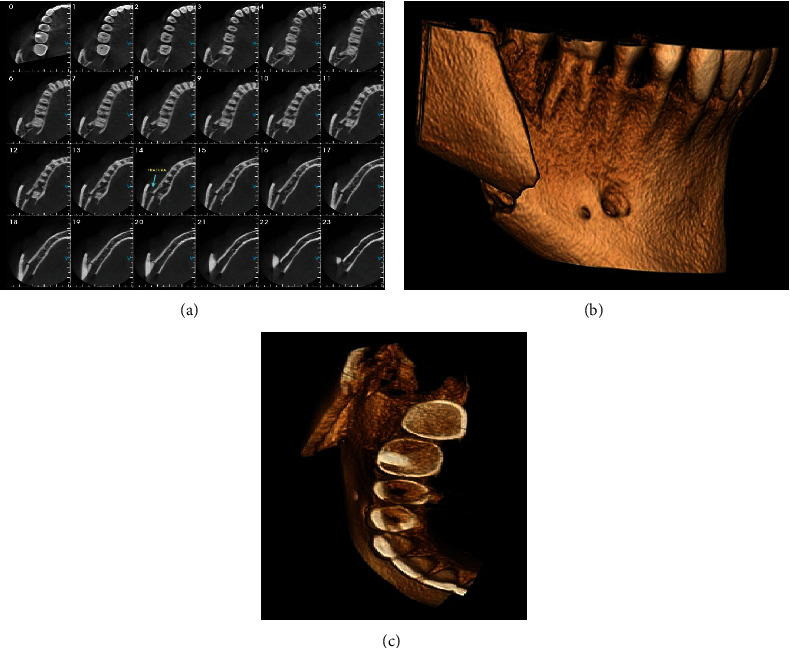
(a) Axial cuts showing right mandibular body fracture. Three-dimensional reconstruction of CT scan showing fracture of the right mandible: (b) lateral view and (c) upper view.
